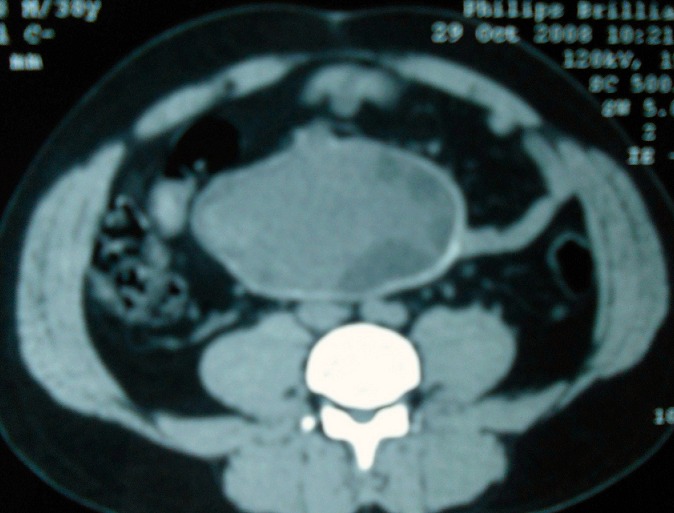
CT scan revealed a large intraperitoneal cyst, with heterogeneous contents
